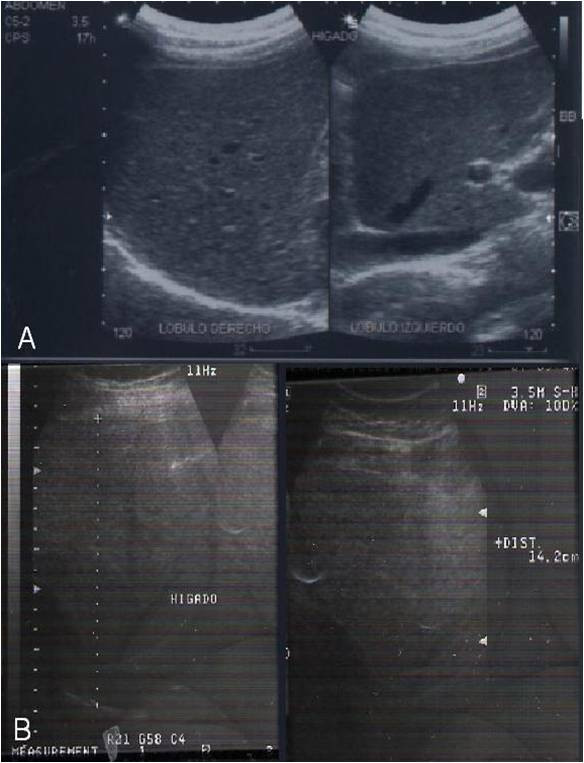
(A) Abdominal ultrasonography of a patient with non-alcoholic fatty liver disease. (B) After a daily administration of Spirulina maxima, a normal liver parenchymal texture could be observed on an ultrasonography.
